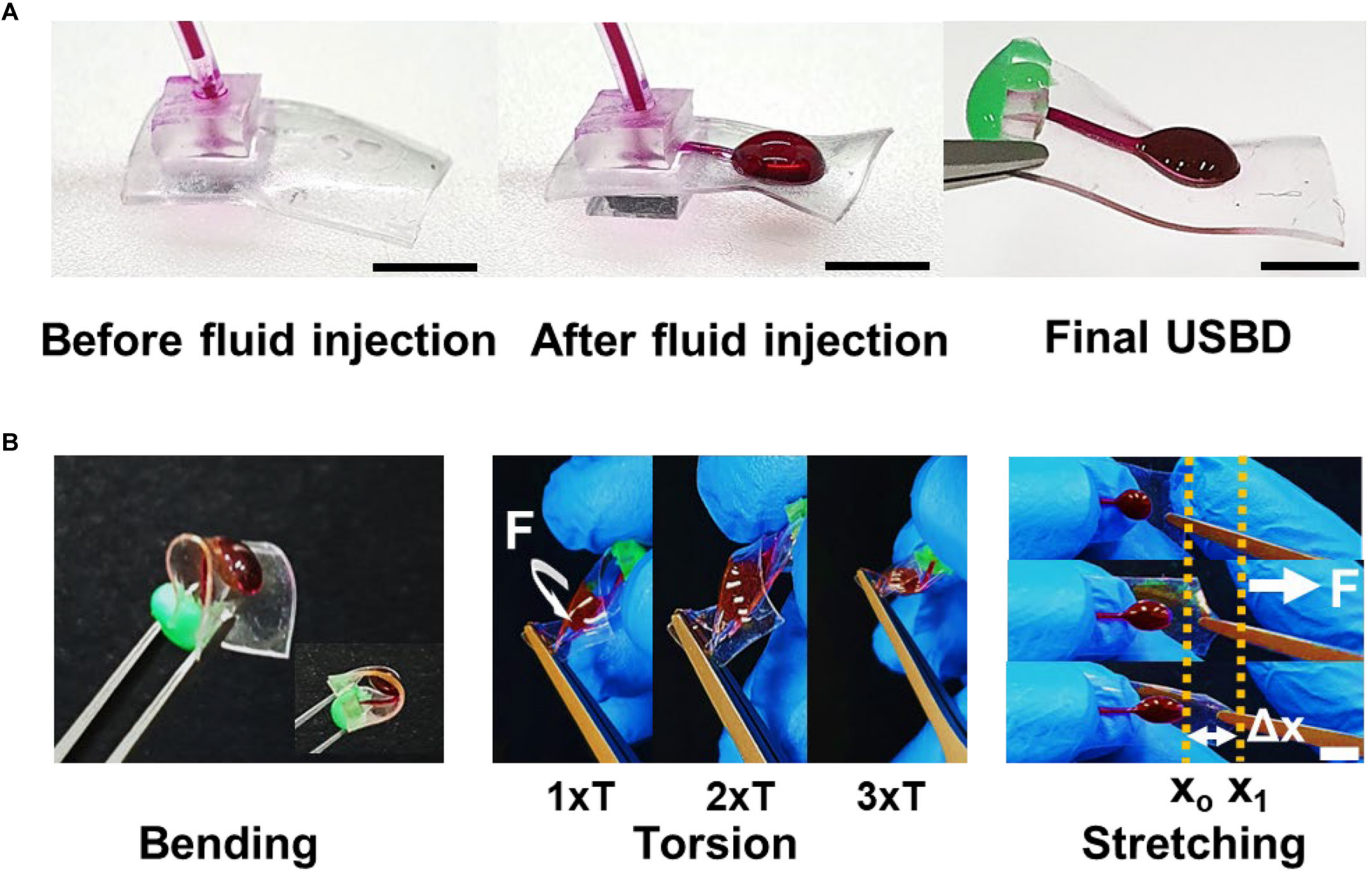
Optical images of the USBD and its softness and flexibility: (A) USBD before and after the injection of RB solution, and (B) images to show its ultrasoft and flexible properties, by applying bending, torsional, and stretching forces. 1xT, 2xT, and 3xT represent the number of twists, and Δ*x* represents the change in length when the USBD was stretched longitudinally, which was 6 mm (35% tensile strain). Scale bars are 5 mm.
